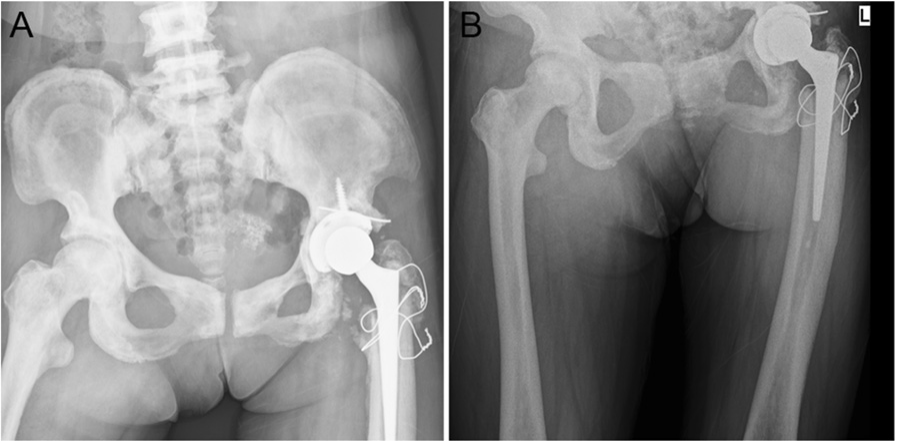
Postoperative radiograph of the left hip. The greater trochanter accidentally broke off and was fixed with steel-wire
